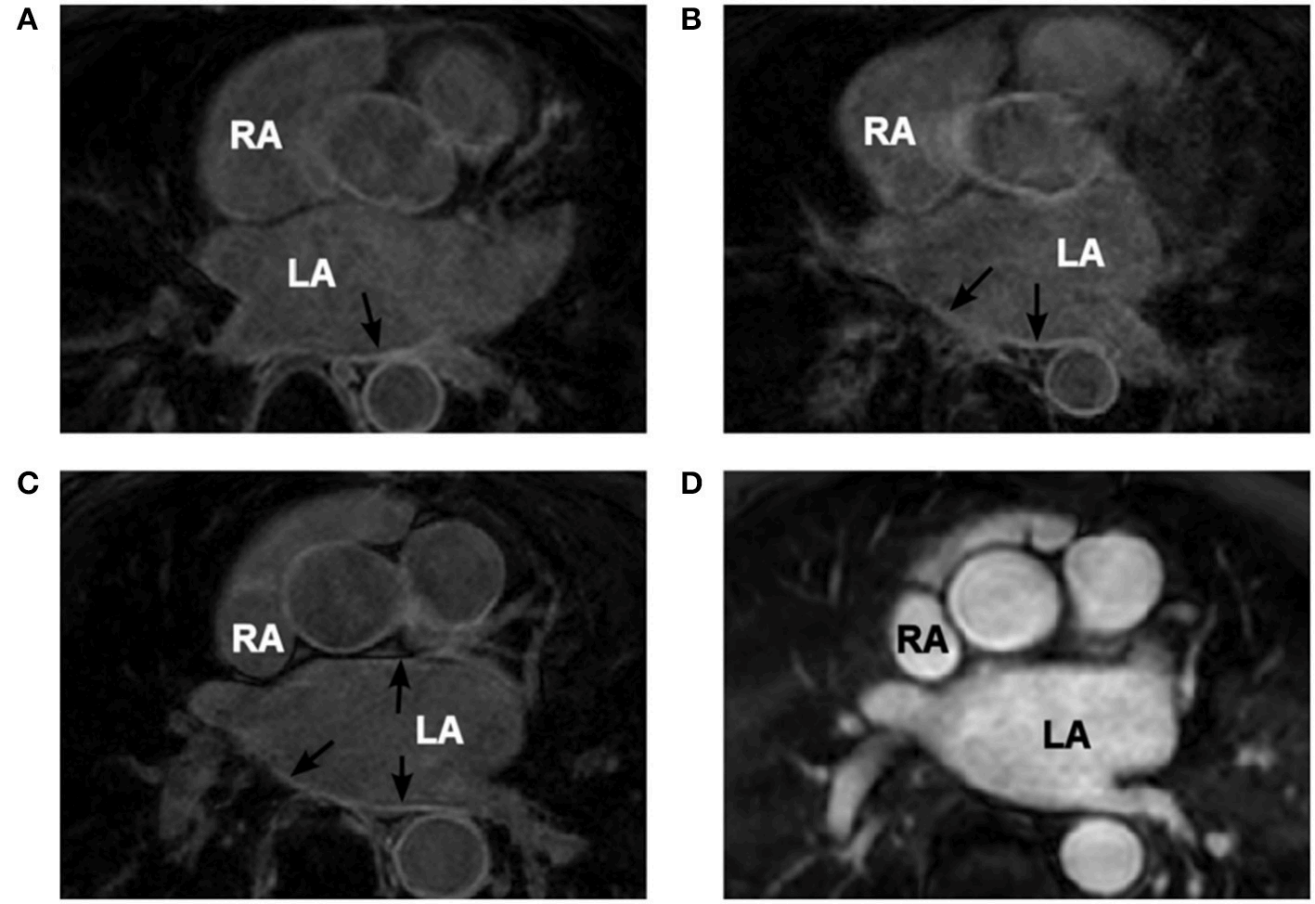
Patient-specific MRI data. (A–C) Coronal LGE MRI slices through the atria (two atria are labelled in white) with areas of high intensity linked to fibrosis indicated by the black arrows. (D) Gd angiographic MR image with high-intensity blood volume, which is used in the segmentation of the endocardial surface. Images in panels (C,D) are from the same patient scan in the same coronal slice.
